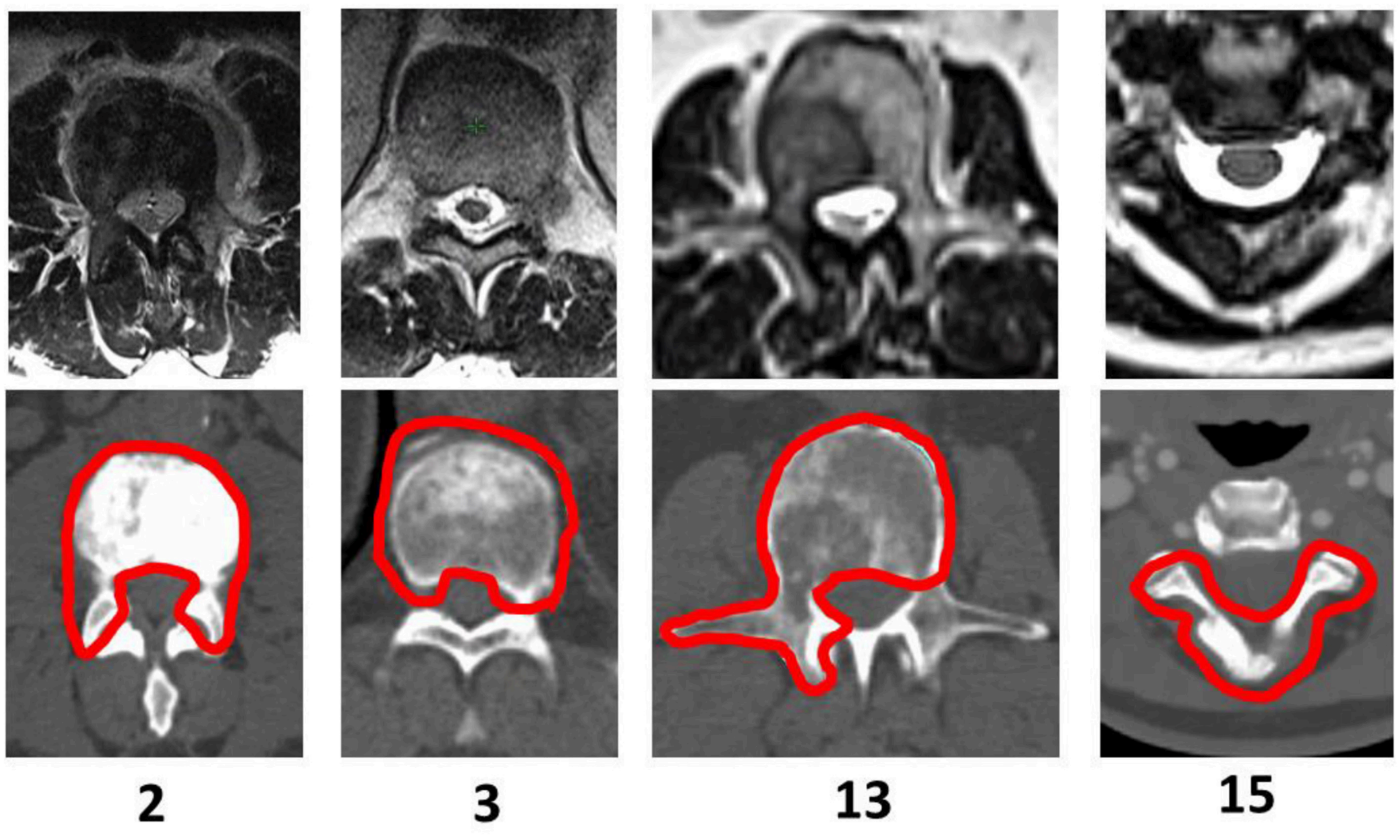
PTV Shapes—Examples of selected patients treated with spine SBRT. The CT slice displayed corresponds to the location of the cord dose maximum for Halcyon™ 1.0 generated treatment plans.
